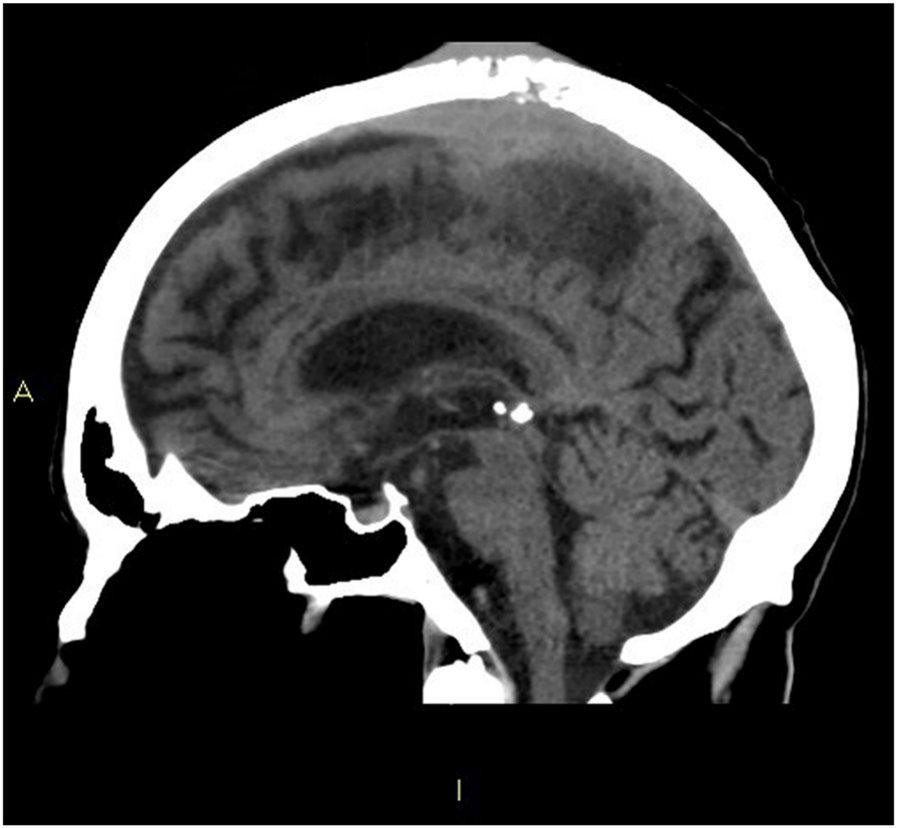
Coronal head computed tomography slice. This image is a coronal slice of the head computed tomography performed on the patient. It demonstrates a single intracranial metastasis with destruction of the parietal bone and extension of the lesion into the extracranial soft tissues.
